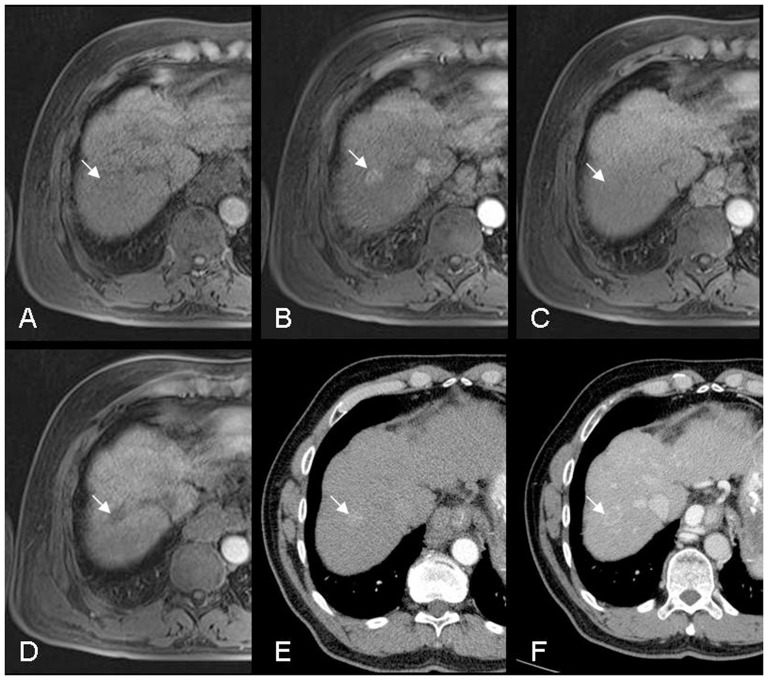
A 59-year-old male patient with liver cirrhosis (Child A) and HCC (arrow) in segment 7. Axial images: A) lesion is barely visible using unenhanced T1w-VIBE, B) marked arterial enhancement in T1w-VIBE following i.v. administration of contrast medium, C) typical washout of the lesion in the equilibrium phase (T1w-VIBE), and D) a clear hypointense lesion in the hepatobiliary phase (20 min after contrast agent injection, T1w-VIBE). Similar behaviour was observed with typical contrast medium enhancement in CT: E) early arterial phase after bolus tracking and F) washout in the portal venous phase with pseudocapsule.
